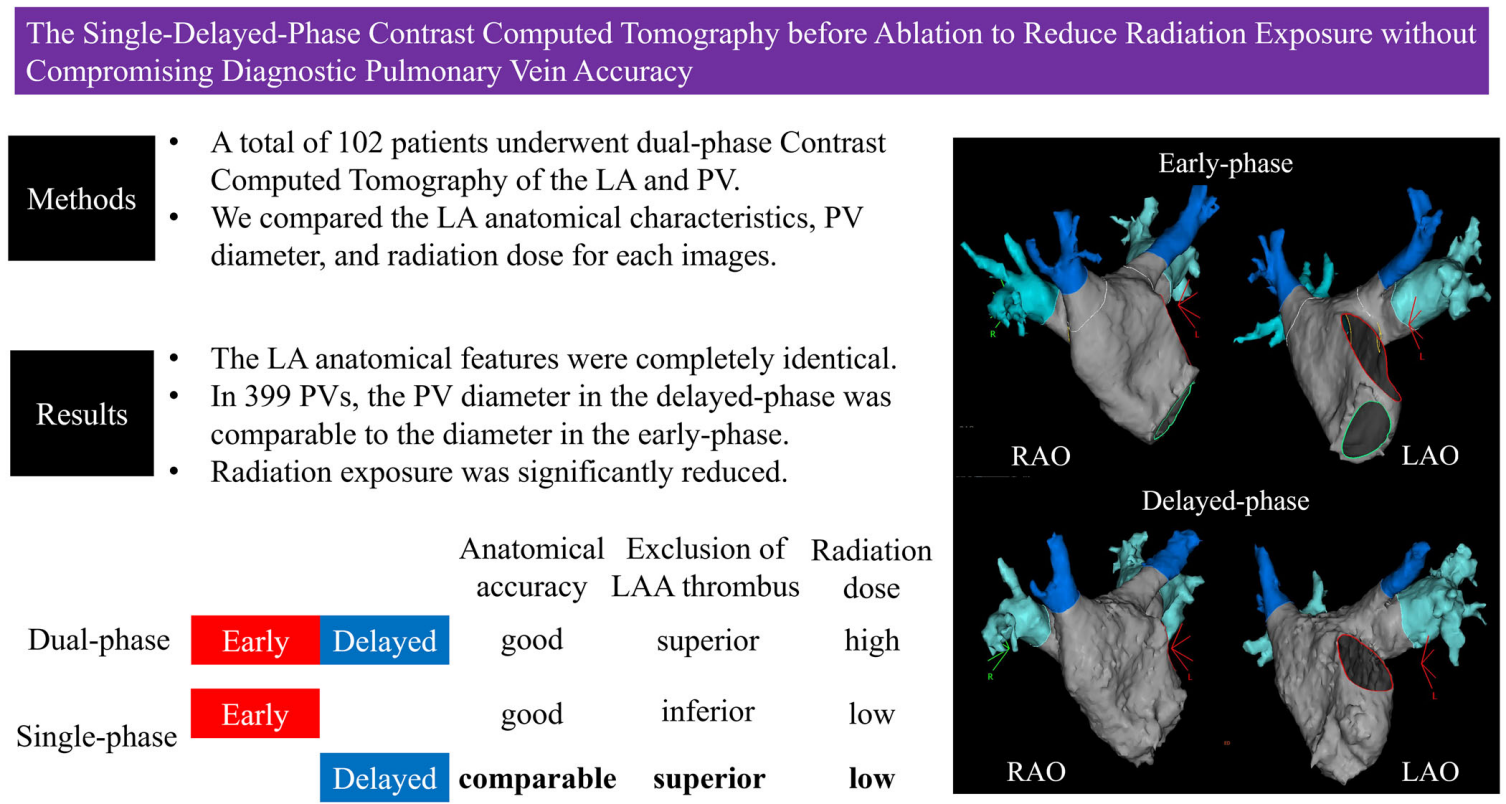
The results of analyzing the early and delayed phases of contrast computed tomography (CCT) images showed that the single‐delayed‐phase was comparable in evaluating the anatomy and diameter of the pulmonary veins to the single‐early‐phase. The single‐delayed‐phase CCT was considered to be effective for evaluation before ablation.
